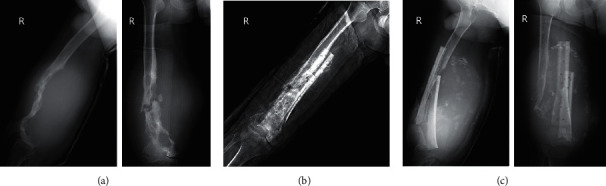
X-ray photography on the right femur of Patient 2. (a) The recurrent hemophilia pseudotumor before the second procedure (internal fixation with an allogeneic bone graft). (b) The postoperative situation of fracture fixation. (c) The collapse of internal fixation and recurrent pseudotumor two years after internal fixation.
